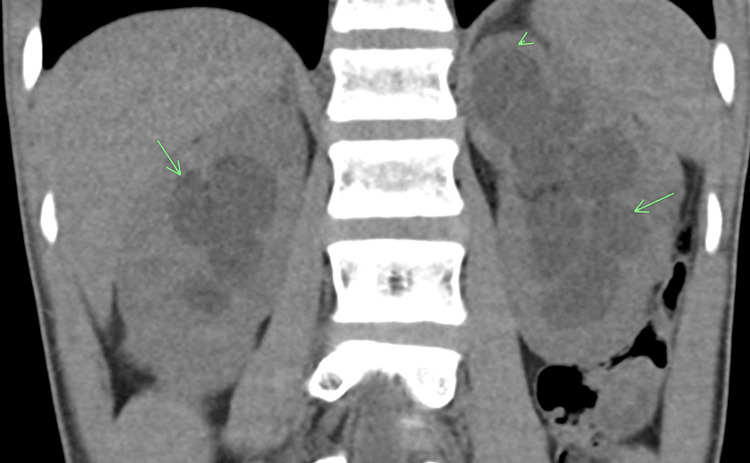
Computer tomography (CT) of the abdomen and pelvis Coronal non-contrast CT image of the abdomen showed bilateral hydroureteronephrosis (green arrows) with left upper pole renal cortical thinning (arrowhead).
